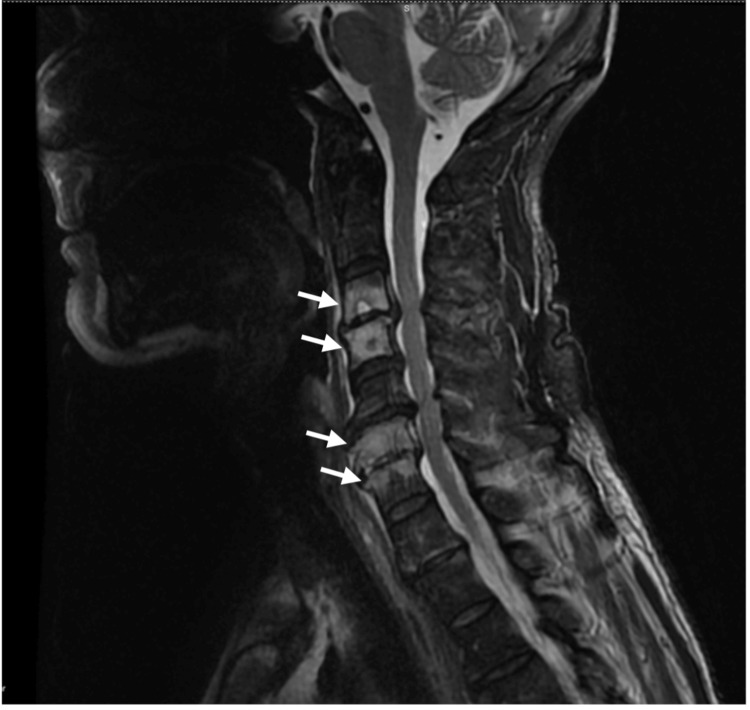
MRI, sagittal T2 of a 57-year-old male with infectious spondilodiscitis of C3, C4, C6, and C7 (white arrows) MRI - Magnetic resonance imaging
